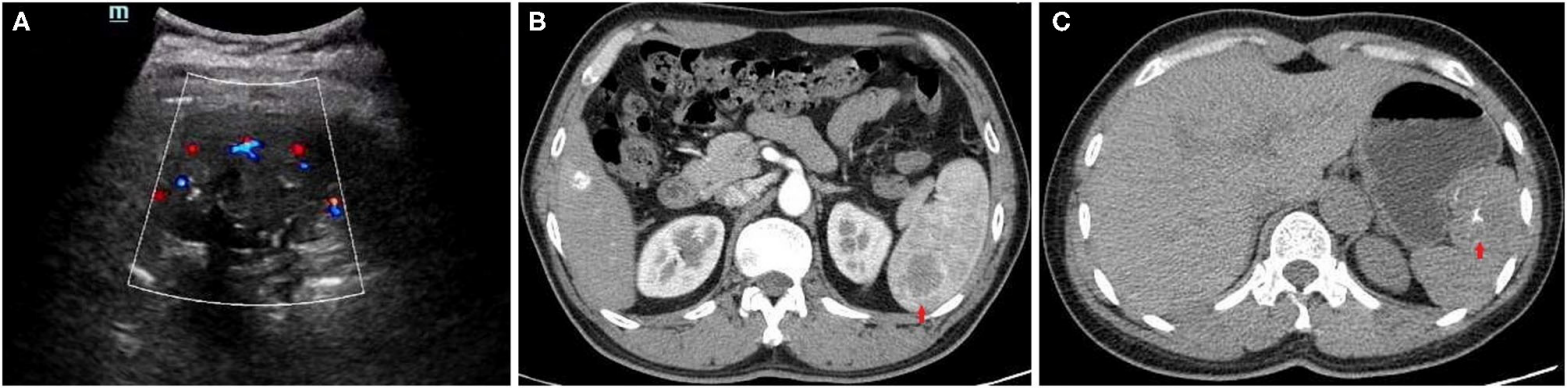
Imaging findings of the SANT patients. (A) US showed a heterogeneous echotexture in the spleen, which was featured by internal vascularity on the color Doppler. (B) A “spoke wheel” pattern and peripheral enhancement around the SANT lesion was seen during the arterial phase. (C) A plain CT scan showed an iso-dense mass with central calcification in the spleen.
